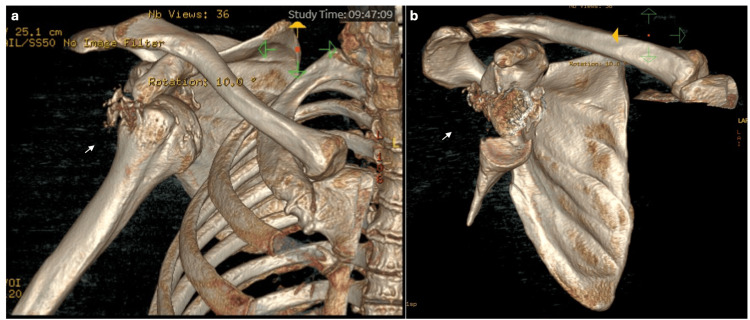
3D reconstruction of right CT shoulder imaging. a. 3D reconstruction showing the anterior view of the glenohumeral joint. Imaging shows a complex comminuted fracture of the glenohumeral joint and posterosuperior fracture of the humeral head. b. 3D reconstruction from CT shoulder, humeral head removed to better view the glenoid. The image displays evidence of a complex comminuted fracture at the anteroinferior aspect of the glenoid with involvement of the intra-articular surface (as shown by white arrows).
